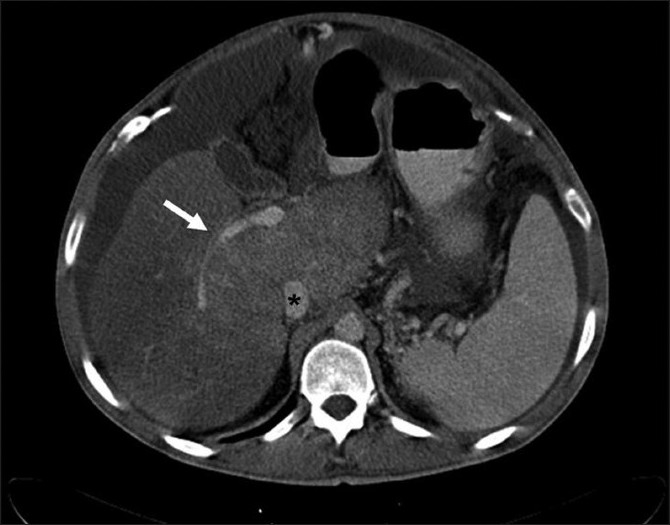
Portal venous phase image of the abdominal CT series of patient number 3 showing the relation between the right portal vein (white arrow) and inferior caval vein (*). Also note that the course of the percutaneous needle was more-or-less similar to the course of the arrow in the caudacranial direction. 187×187mm (150 × 150 DPI)
